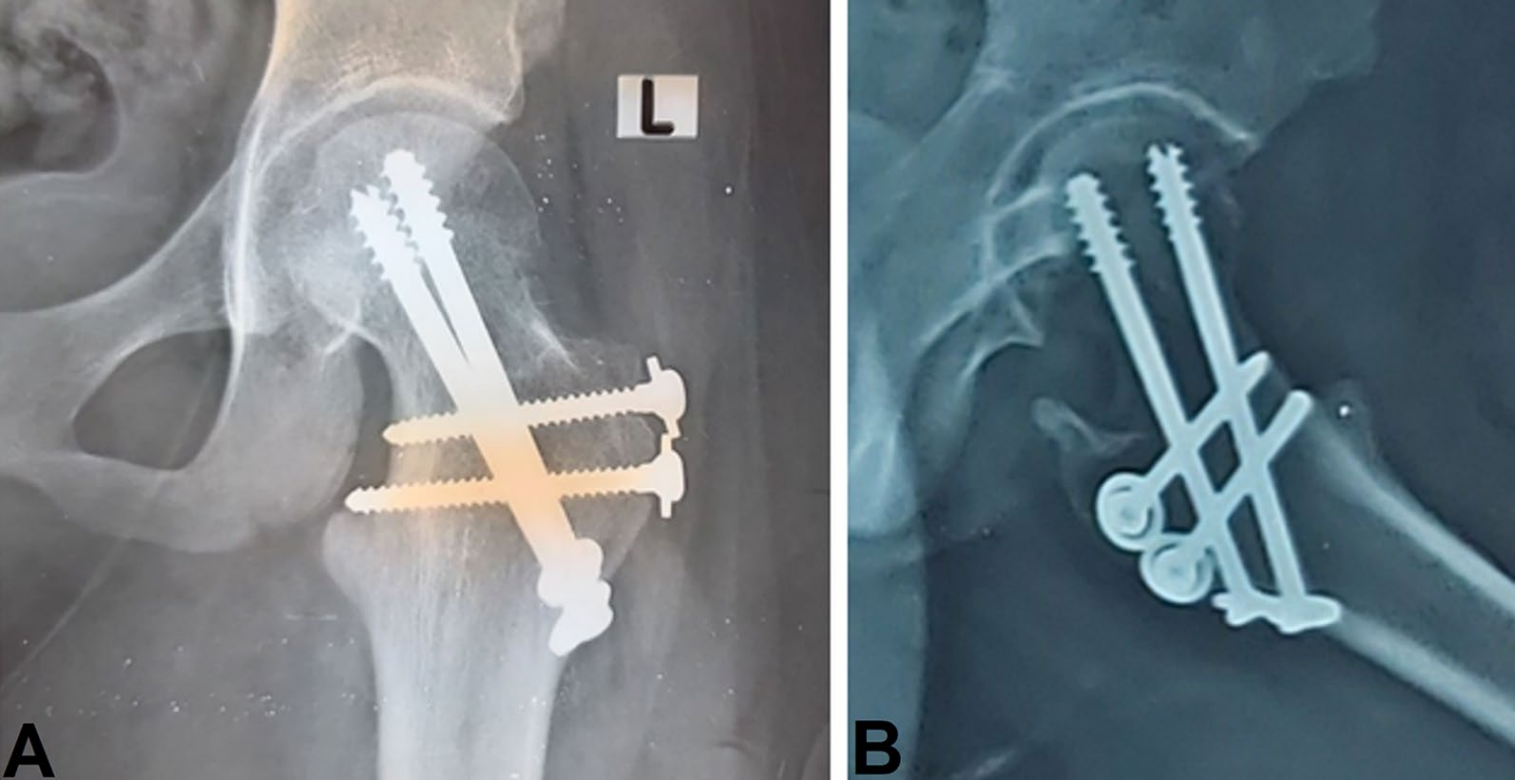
Six-year follow-up X-rays. A AP view. B Lateral view
